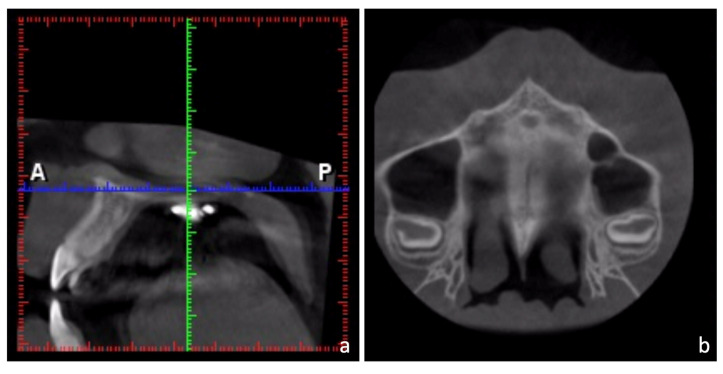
Examination of the maturation stage of the midpalatal suture on pre-expansion CBCT images by applying the method of Angelieri et al. [28] (a) Head orientation was done on the CBCT images. (b) The axial plane was set in coincidence with the palatal plane and the maturation stage of the midpalatal suture was in stage C.
